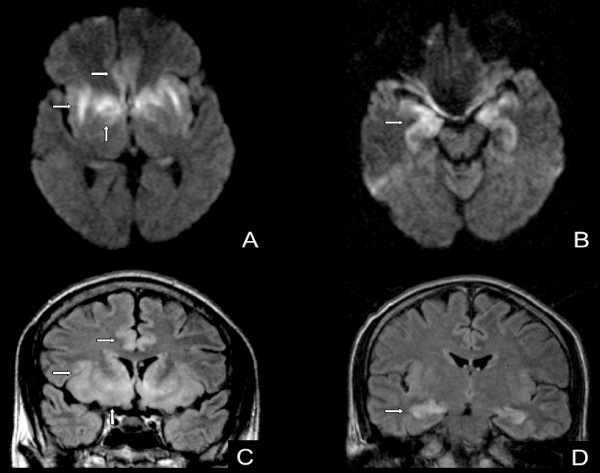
MRI of the brain: Typically bilateral and asymmetric encephalitis of the limbic system. Diffusion weighted images (A + B; axial view): restricted diffusion (hyperintense signal) in the cingulate gyri, insula, and temporal lobes (arrows). FLAIR (C + D; coronal view) sequences: hyperintense swollen cortex and subcortical white matter (arrows) in the medial temporal lobes and cingulate gyri.
